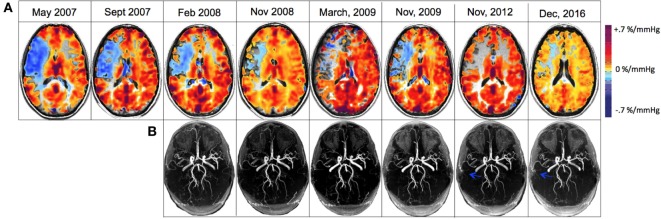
(A) Cerebrovascular reactivity maps overlaid on anatomical 3D T1-weighted acquisitions for registration to MNI space for displaying matching coordinates for region analysis. (B) 3D time-of-flight magnetic resonance angiography (MRA) of the circle of Willis that were re-registered to one another and slice corrected to include only the identical slices within the brain slab for each acquisition. Blue arrows denote the new collateralized vessels compared with previous scans.
